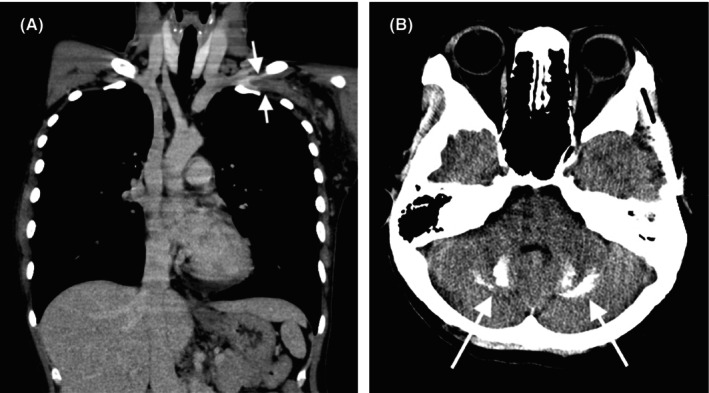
Contrast‐enhanced computed tomography of the best revealed thrombosis extending from the left axillary to the subclavian vein (A, arrow), with significant venous obstruction. Concurrently, plain computed tomography of the brain identified calcifications with the bilateral cerebellar dentate nuclei and globus pallidus (B, arrow), hallmark features suggestive of neuropsychiatric systemic lupus erythematosus.
